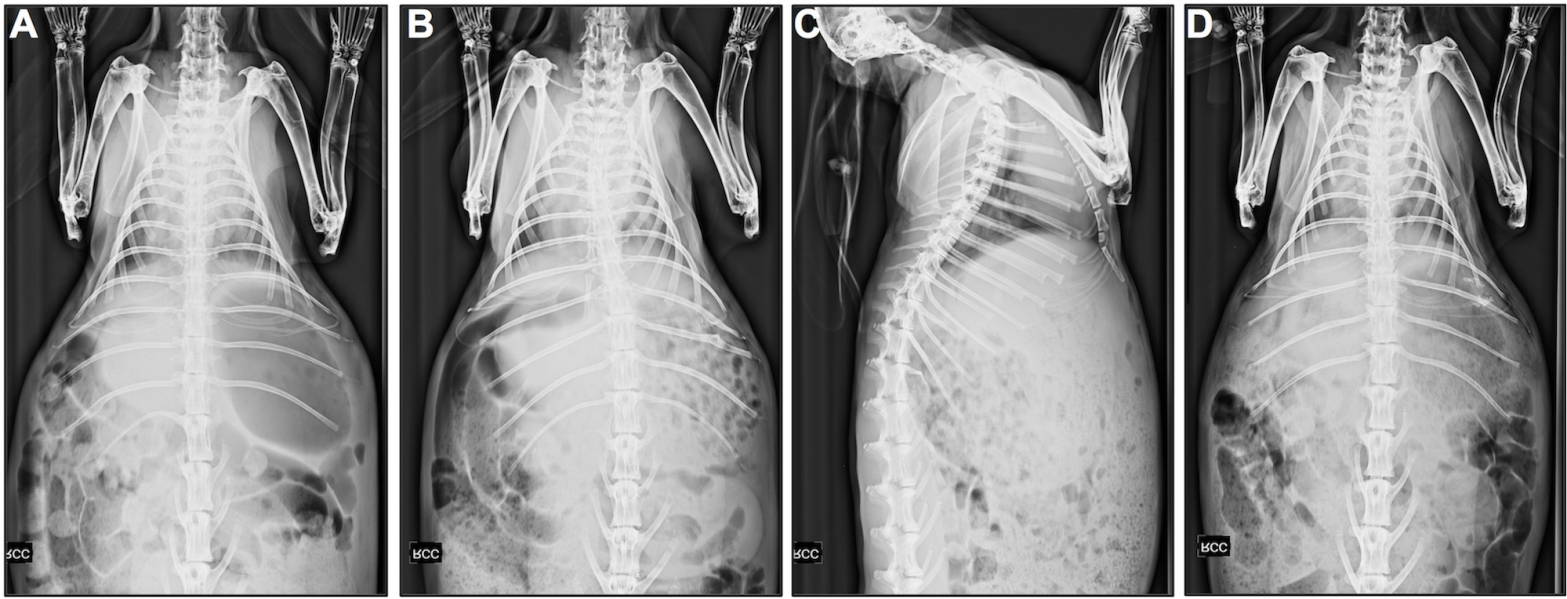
Radiographic evaluation. Unoperated control (d90; A), left-convex scoliosis, postoperative changes of the 9^th^ and 10^th^ left rib (SIS d90; B), discrete elevation of the left diaphragm (SIS d60; C), and discrete reduced left-thoracic volume with postoperative calcifications adjacent to the 8^th^ left rib (Matricel d90; D).
